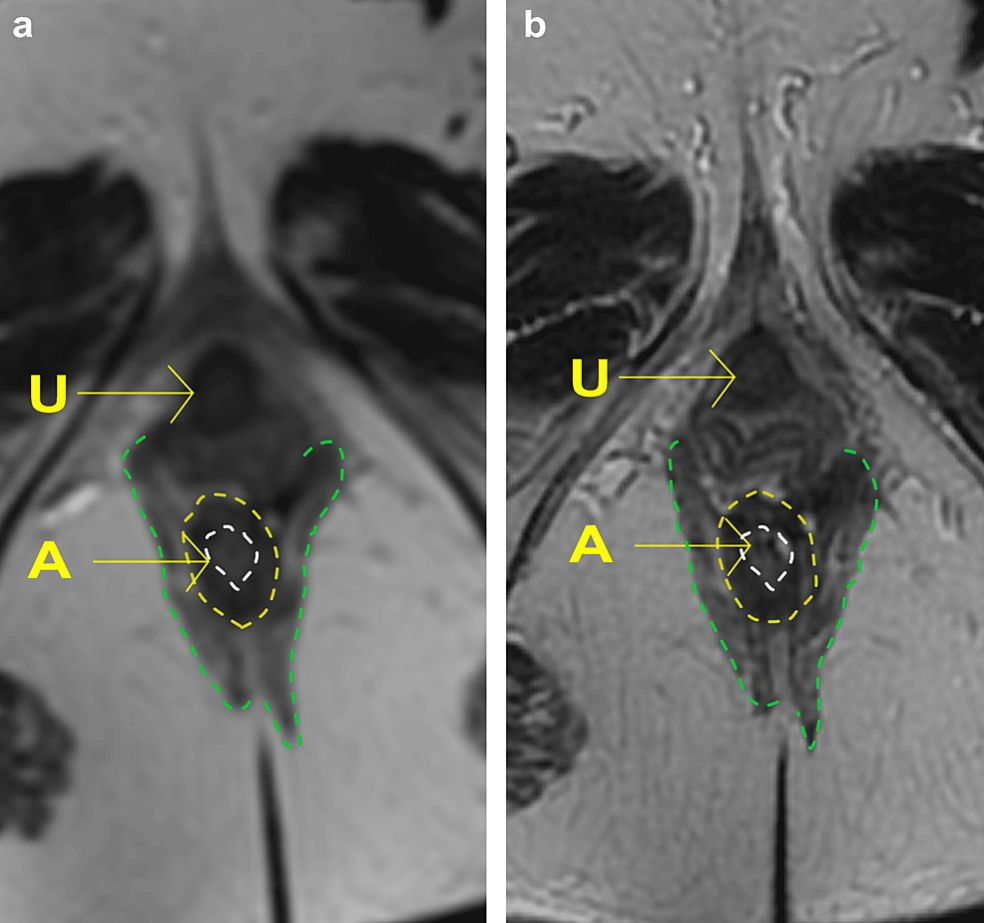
The anal sphincter muscles The T2-weighted sagittal view MRI using a 1.5T MRI (Signa Creator, GE Healthcare, Chicago, USA). The images, with specified MRI parameters, show a comparison before (a: T0) and after (b: T18) treatment, with the urethra (U) and the anus (A) indicated by arrows. The yellow dotted lines identify the internal anal sphincter as seen on MRI, the green dotted lines represent the external anal sphincter, and the white lines outline the contour of the anus.
